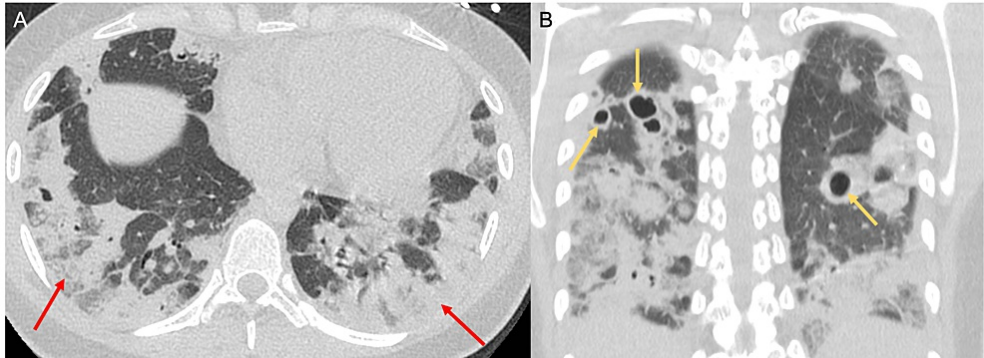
Axial (A) and coronal (B) CT images of the chest in the lung window demonstrate dependent ground-glass opacities involving bilateral lower lobes (red arrows in A). Cavitary lesions are present throughout the lungs (yellow arrows in B).
